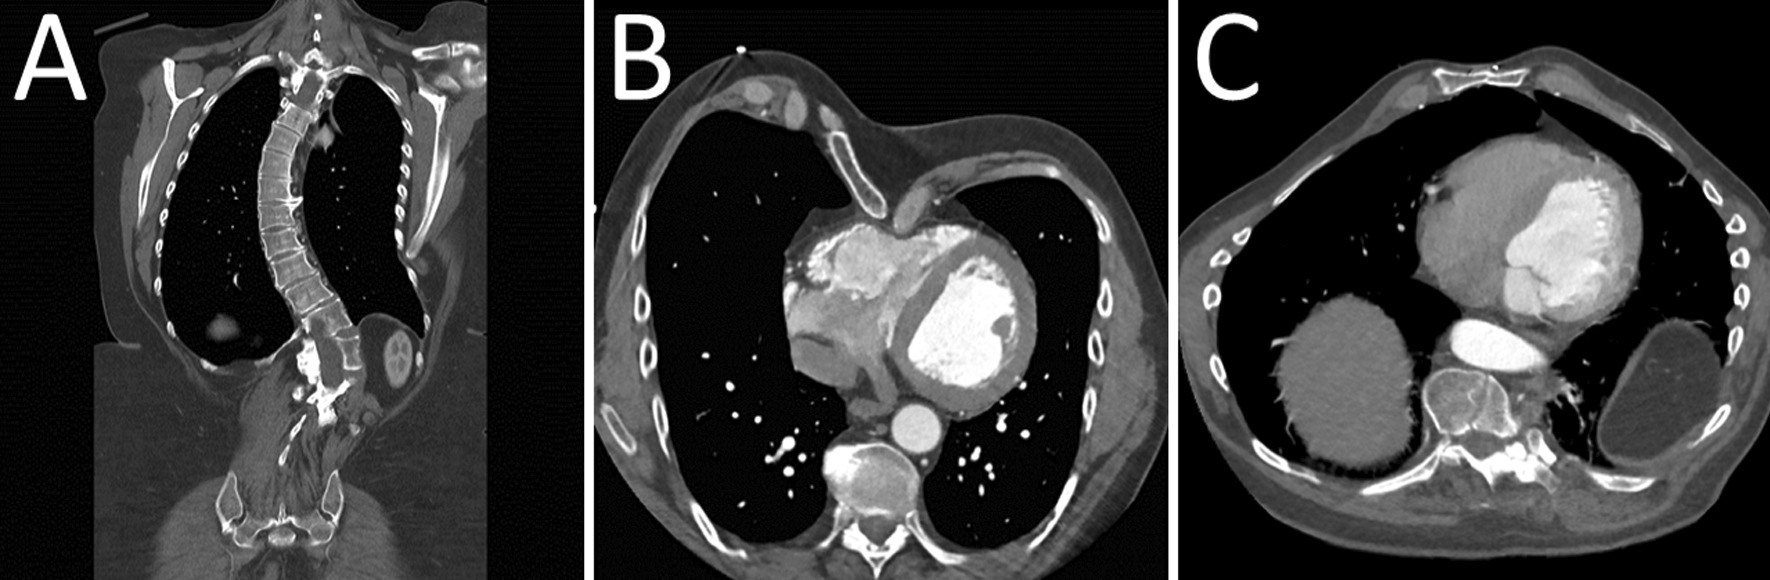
Skeletal abnormalities in two adults with Marfan syndrome. A 37-year-old women with new diagnosed Marfan syndrome. CT scan reveals a thoracic dextroscoliosis with osteophytes. B 56-year-old male with Marfan syndrome and severe pectus excavatum with compression of the right ventricle. Even though the patient was diagnosed as a child he was never offered corrective surgery of the chest. C 38-year-old male with pectus carinatum. He had never been interested in corrective surgery
